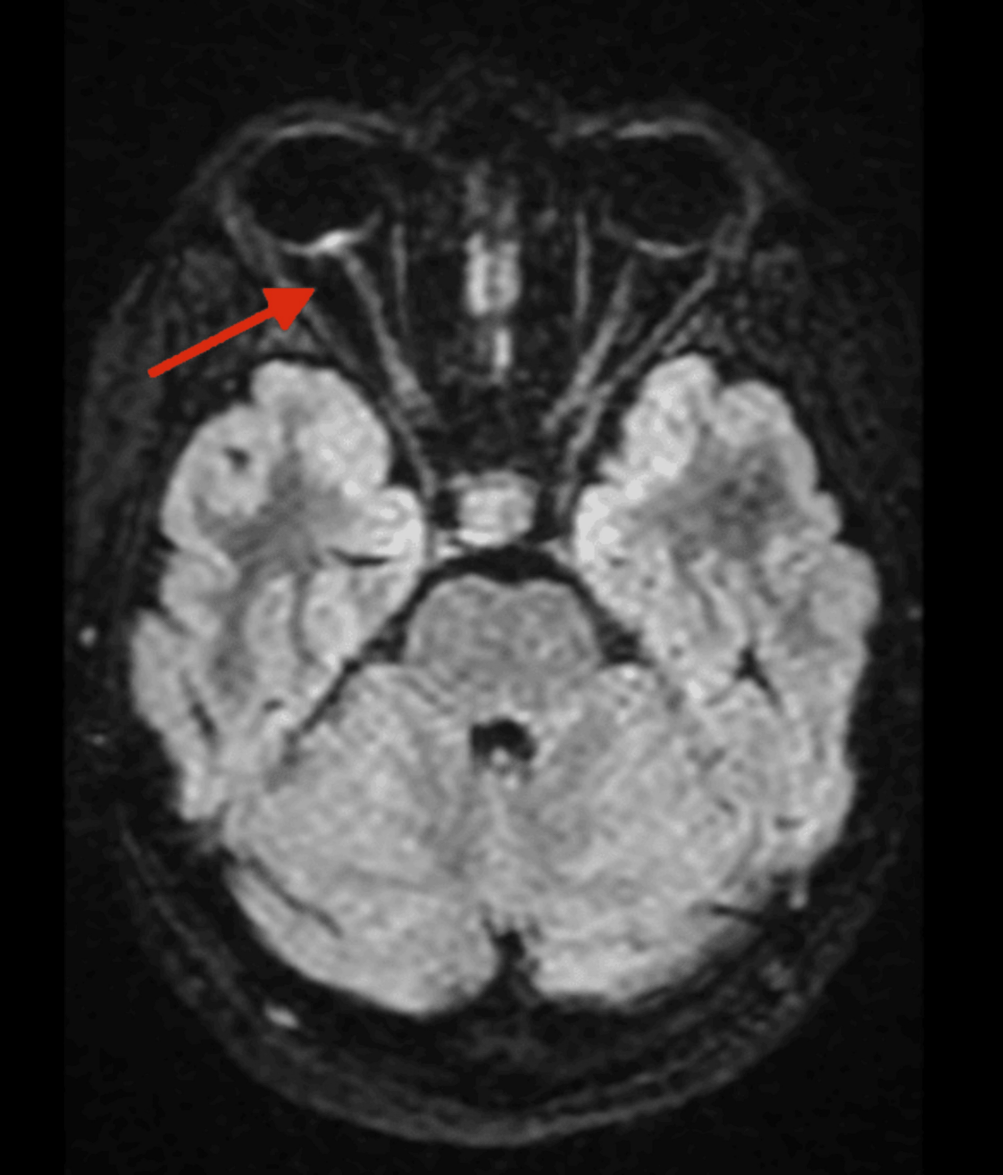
MRI findings Axial and coronal T1-weighted postcontrast fat-suppressed images showing marked enhancement and thickening of the right intraorbital optic nerve, consistent with acute inflammatory neuritis. The left optic nerve, chiasm, and brain parenchyma are normal, with no demyelinating or ischemic lesions
